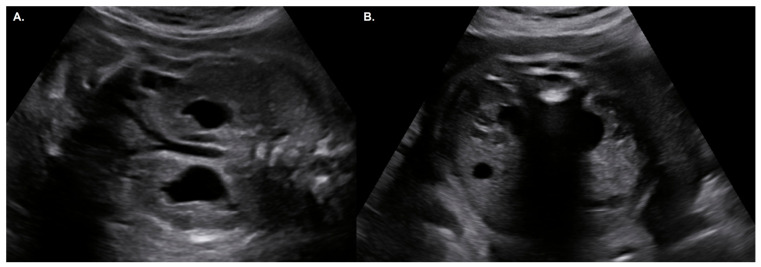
A representative case from Group 2: prenatally detected renal abnormalities without recognition of HSK. Prenatal ultrasonography at 29 weeks of gestation demonstrating bilateral hydronephrosis. While renal pelvis dilatation was identified, the underlying fusion anomaly was not definitively diagnosed during the prenatal period. (A) Coronal view and (B) Axial view of gray-scale image showing dilated renal pelvis. HSK, horseshoe kidney.
